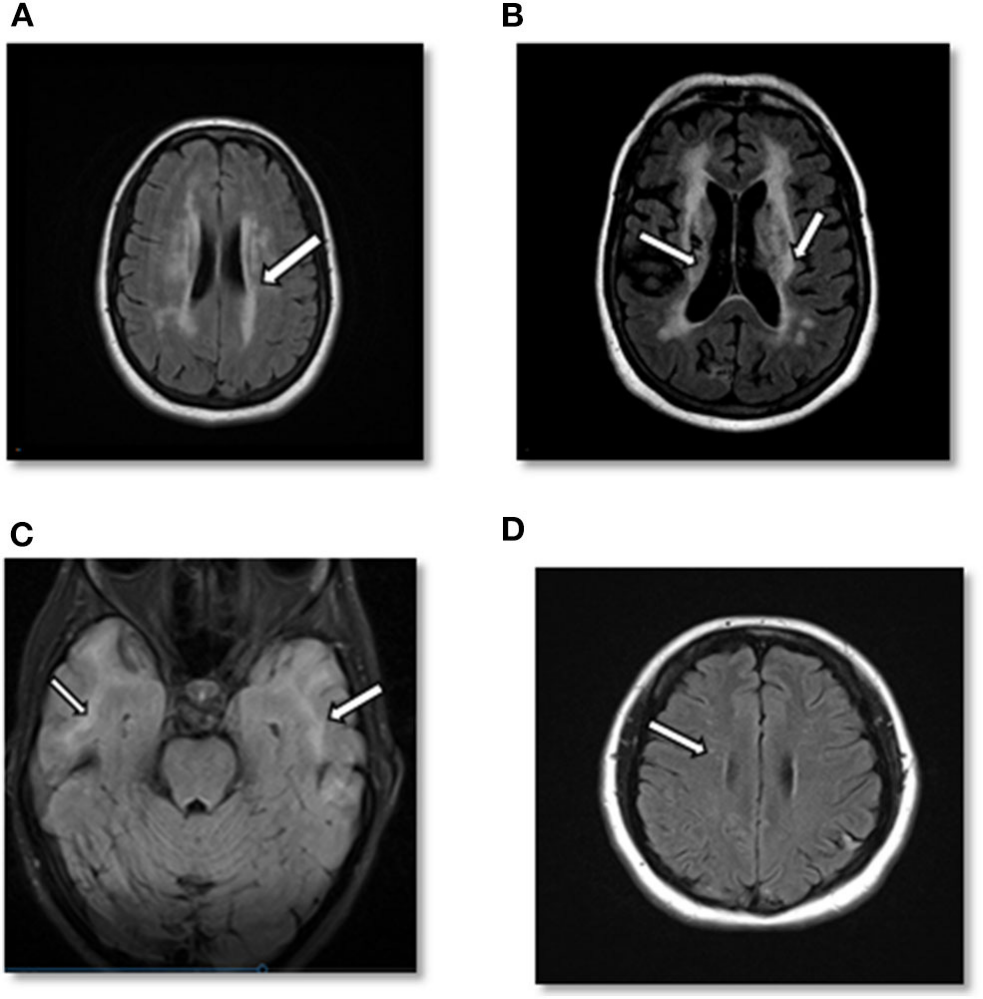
(A) Case 1: Axial cerebral T2-FLAIR image demonstrates T2 hyperintense signal in periventricular area. (B) Case 1: Axial T2-FLAIR image after 10 years demonstrates significant worsening of T2 hyperintense signal in periventricular and subcortical areas with multiple prominent lacunar infarcts as well as cortical atrophy. (C) Case 2: Axial cerebral T2-FLAIR showing confluent white matter hyperintensities in bilateral temporal lobes. (D) Case 3: Axial cerebral T2-FLAIR: small foci of T2-FLAIR hyperintensity in the frontal lobe.
